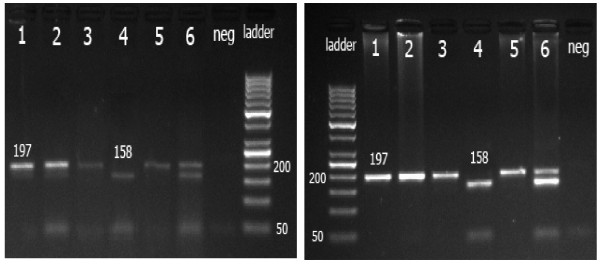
Gel electrophoresis of C3435T polymorphism from tissue samples. Left: The last lane from the right is 50 bp DNA ladder. Samples in lanes 1, 3 and 5 represent the PCR products and samples in lanes 2, 4 and 6, are the digest products of each sample, respectively. Sample in lane 2 is the mutant homozygous uncut TT genotype (197 bp). Sample in lane 4 represents the wild type cut CC genotype (158 bp and 39 bp). Sample in lane 6 represents heterozygous CT genotype (197 bp, 158 bp and 39 bp). Right: Gel electrophoresis of C3435T polymorphism from blood samples. The first lane from the left is 50 bp DNA ladder. Samples in lanes 1, 3 and 5 represent the PCR products and samples in lanes 2, 4 and 6, are the digest products of each sample, respectively. Sample in lane 2 is the mutant homozygous uncut TT genotype (197 bp). Sample in lane 4 represents the wild type cut CC genotype (158 bp and 39 bp). Sample in lane 6 represents heterozygous CT genotype (197 bp, 158 bp and 39 bp).
